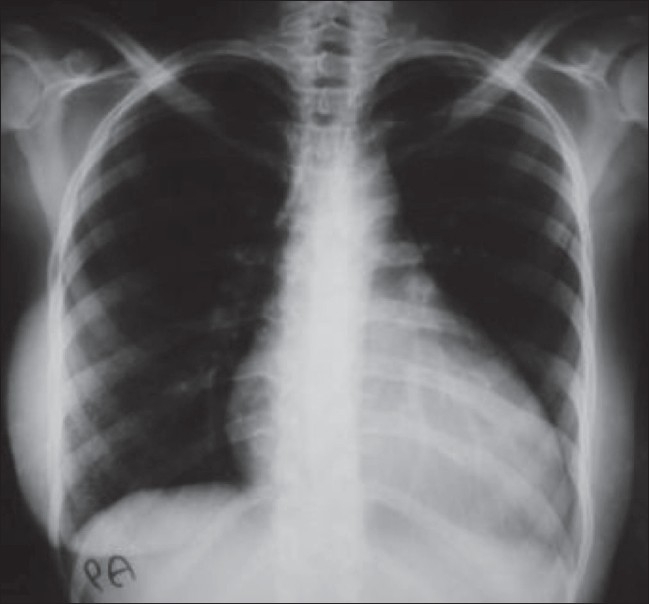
X-ray chest shows diffuse calcifications in both breasts
